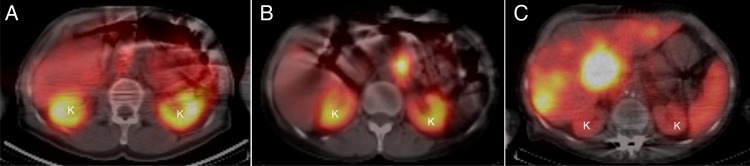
Transversal SPECT/CT midsections of the kidneys (K) in three patients treated with ^177^Lu-DOTATATE. A–C correspond to the same patients investigated with planar image as in Figure 1.
